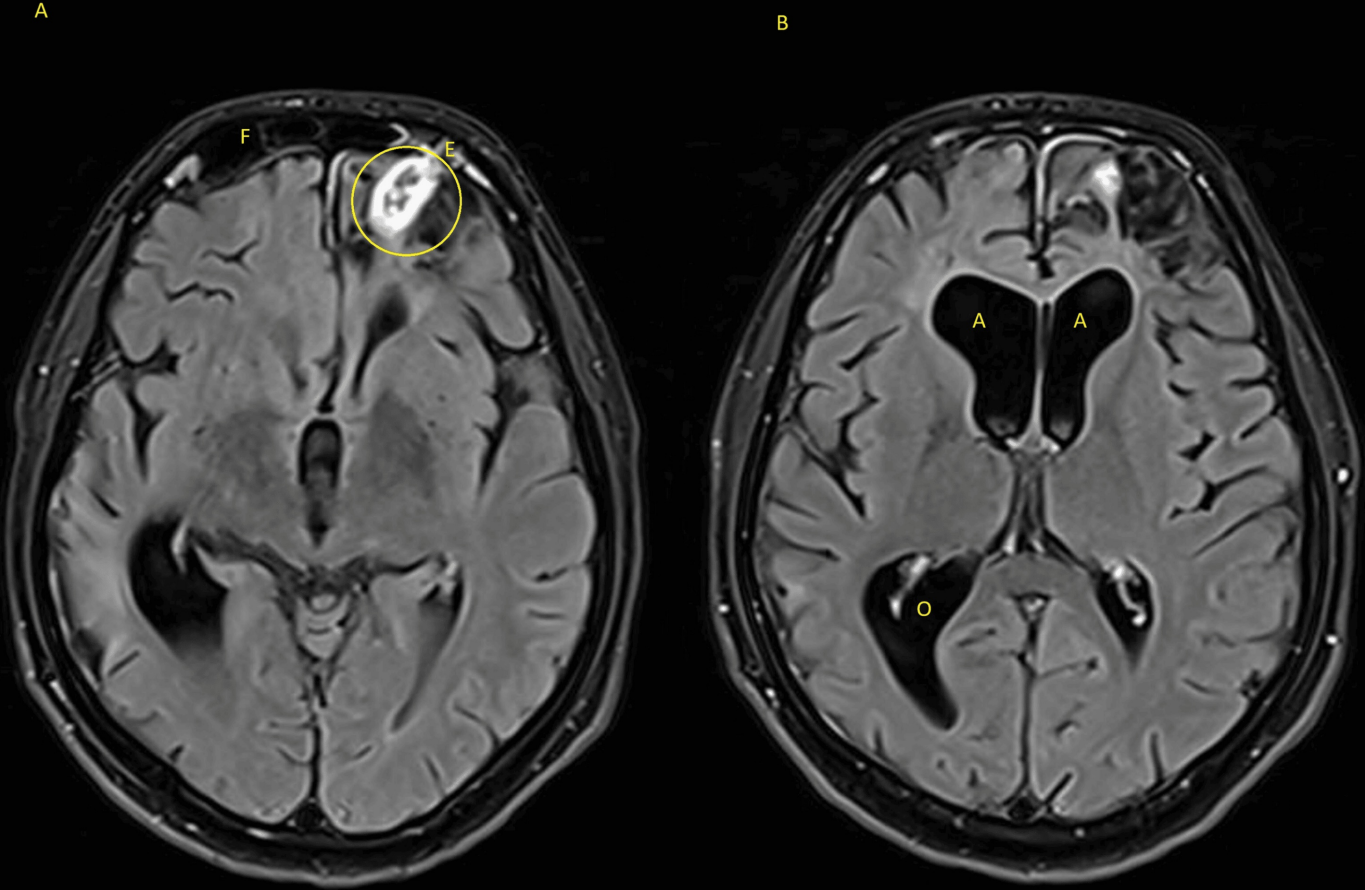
MRI scan’s T1 axial view with contrast showing, in section A, a ring-enhancing collection (circle) in the left frontal lobe, and an encephalocele (E) in the frontal sinus (F). Section B shows hydrocephalus in the anterior (A) and occipital (O) horns of the lateral ventricle.
